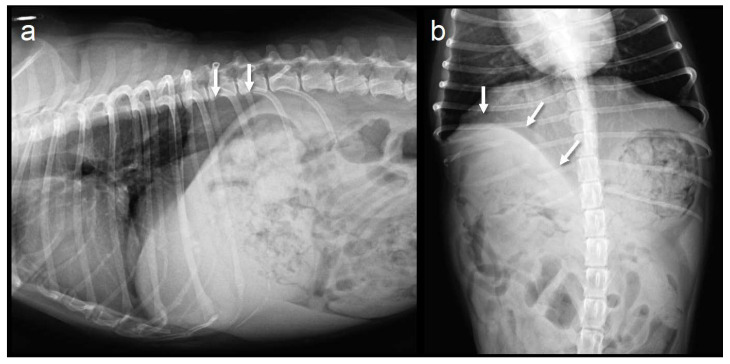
Abdominal radiographs at the 6-month follow-up. The suture line of the diaphragm and thoracic wall is visualized (arrows). (a) Lateral view; (b) Ventrodorsal view.
